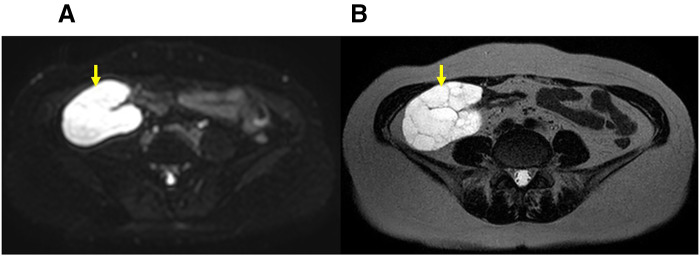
MRI findings. (A) Diffusion-weighted image of the tumor. (B) T2-weighted image of the tumor. MRIs show multiple cystic lesions with clear boundaries, numerous septa, and no solid components. A clear boundary is observed between the lesions and the ascending colon. The diffusion-weighted image shows homogeneous, high signal intensity within the tumor. The yellow arrow indicates the tumor.
